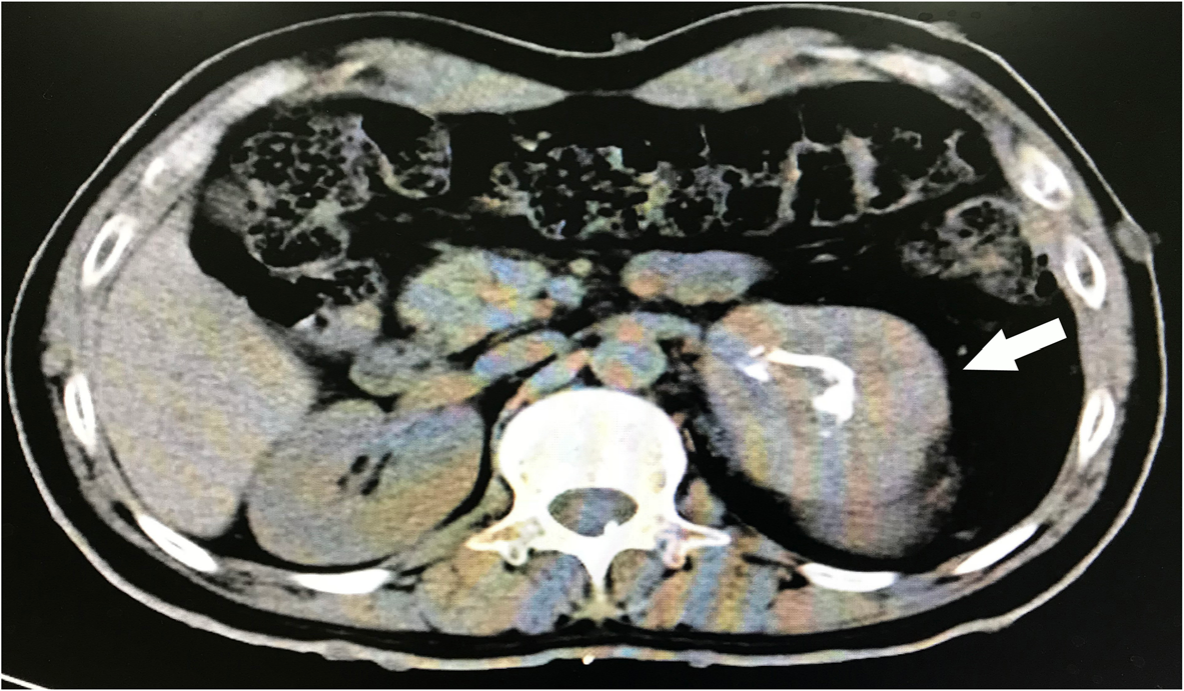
Enhanced CT of abdomen demonstrated a 7.7 cm × 6.7cm heterogeneous mass in the left adrenal gland (white arrow)
